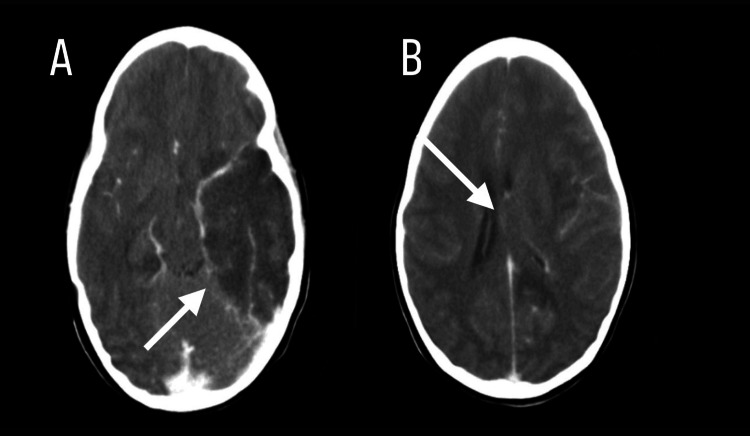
1A: CT showing extensive ischemic reaction in the left temporal lobe ( indicated by the white arrow). 1B: CT performed showing collapse of the ventricular system (indicated by the white arrow).
